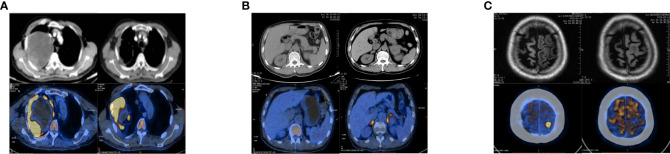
Changes in chest and upper abdomen contrast-enhanced CT, PET-CT, and brain MRI before and after treatment. (A) Baseline (left): Chest CT showed a space-occupying mass in the right upper lobe (10.6 × 8.5 cm), and the possibility of lung cancer was considered. PET-CT showed a large soft tissue mass with necrosis in the right upper lobe (12.2 × 11.3 × 12.6 cm) with increased annular metabolism (SUVmax: 27.3). Follow-up after 9 months of treatment (right): Chest CT showed that the lesion in the right upper lung lobe had become smaller (7.8 × 6.0 cm). PET-CT showed that the wall of the nodule and the solid component of the mass in the right upper lobe were smaller than before (6.8 × 9.0 × 7.9 cm), had a slightly lower SUVmax (25.7), involved the right chest wall, and partially extended into the right axilla. (B) Baseline (left): Abdominal CT and PET-CT showed no obvious abnormality of the adrenal gland. Nine months after treatment (right): Abdominal CT showed that the left adrenal nodule was enlarged and that a new nodule had formed in the right adrenal gland. PET-CT showed bilateral adrenal soft tissue masses; the larger one was located on the left side and measured about 4.1 × 3.6 cm with an SUVmax of 9.9 to 11.2. (C) Baseline (left): Brain MRI showed a left anterior central gyrus nodule (approximately 1.0 cm in diameter), and metastasis was considered. PET-CT showed a slightly dense nodule at the junction of the cortex and medulla in the left frontal lobe (1.1 × 1.4 × 1.0 cm) with an SUVmax of 26.2. Nine months post-treatment (right): Brain MRI showed that the left anterior central gyrus nodule was slightly smaller than the anterior one (about 0.9 cm in diameter), and the post-treatment inhibition of the activity of the metastatic focus was considered. PET-CT showed a slightly dense nodule (diameter of 0.8 cm) at the junction of the cortex and medulla of the left frontal lobe (SUVmax: sparse radioactive distribution). CT, computed tomography; PET-CT, positron emission tomography-computed tomography; MRI, magnetic resonance imaging; SUVmax, maximum standardized uptake value.
